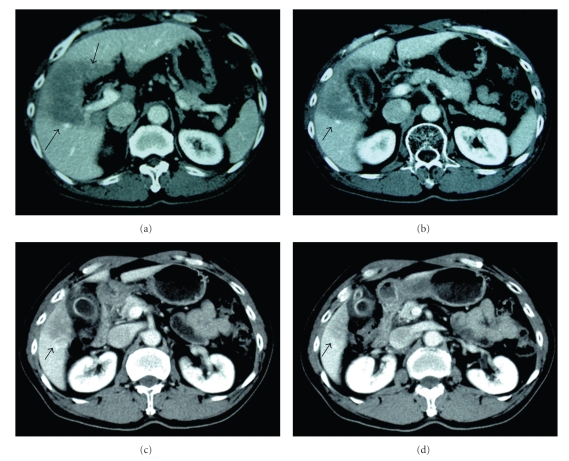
Postradiation CT scans corresponding to the sections in Figure 1. The region of low attenuation in the liver (arrows) corresponded to the treatment volume at 2 months after completion of 3D CRT. The tumor appears to have a complete response to 3D CRT.
